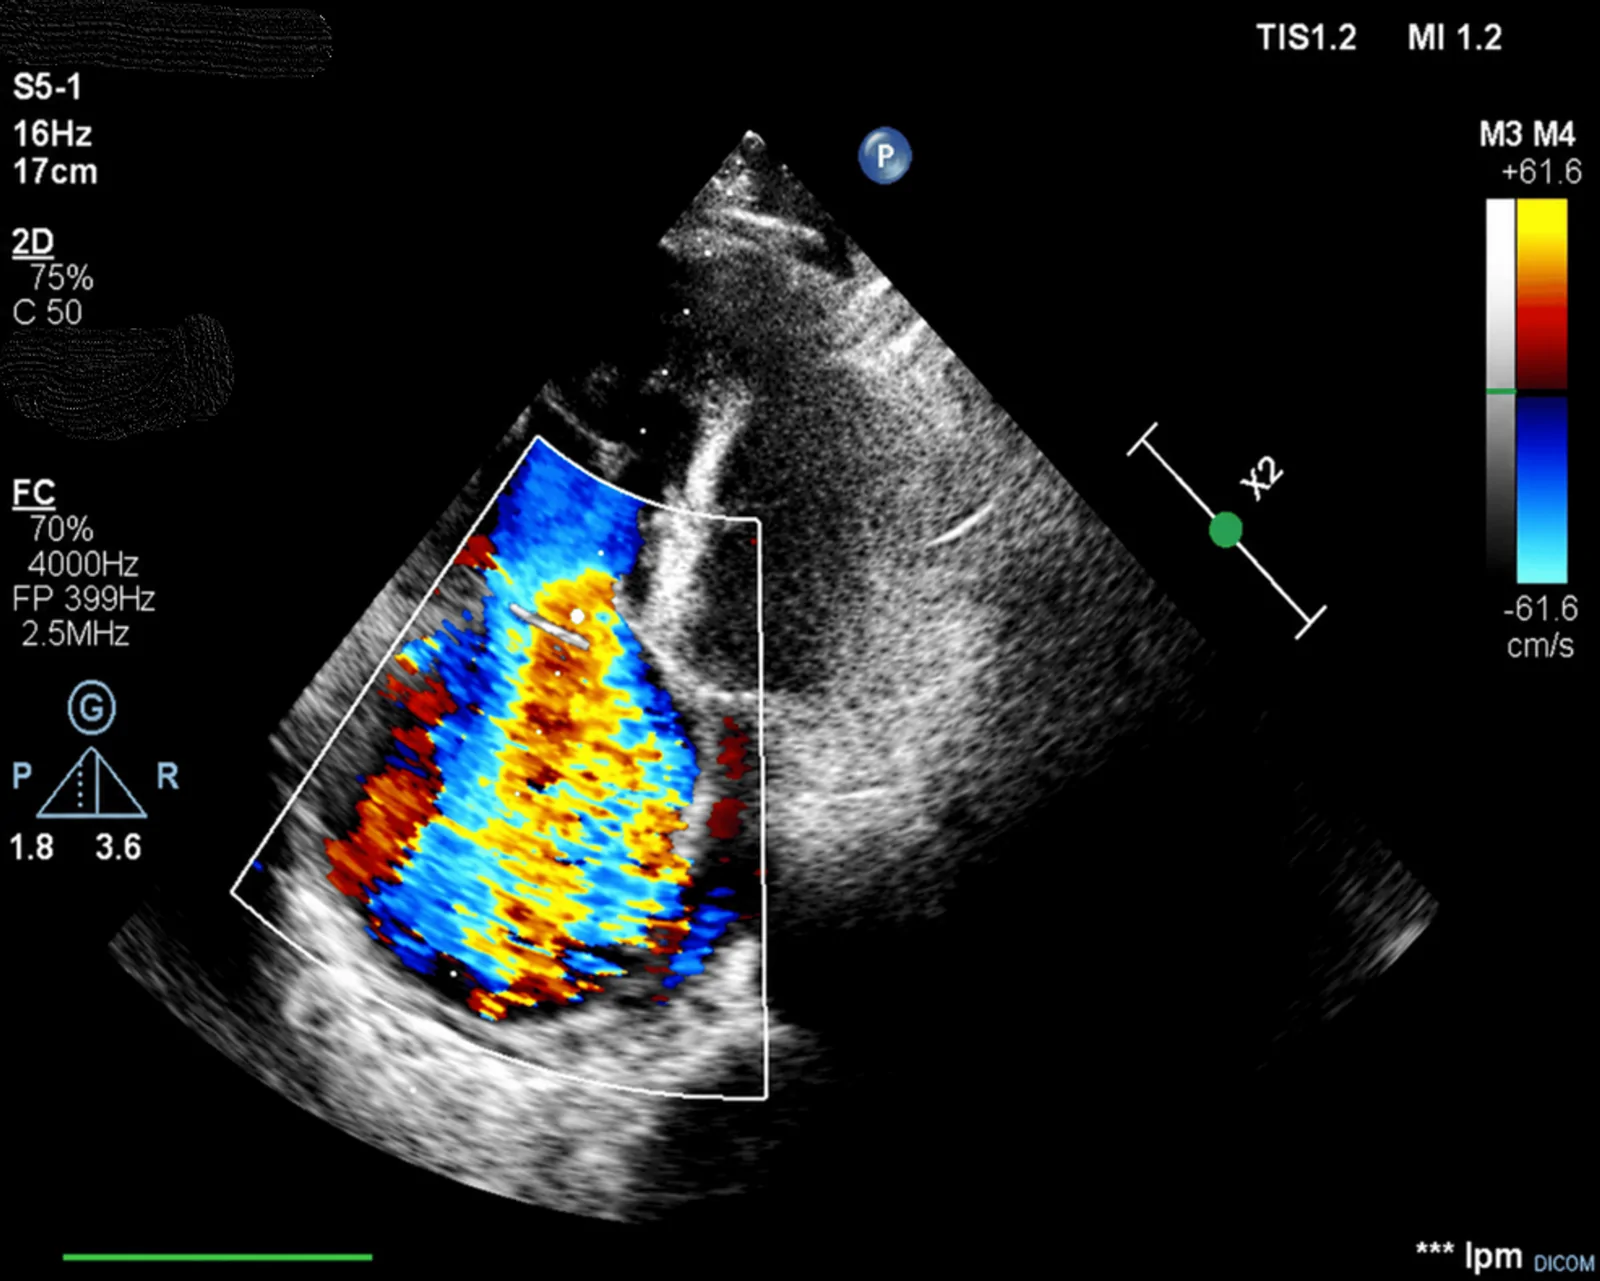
Transthoracic echocardiogram showing enlargement of the tricuspid annulus with tethered leaflets and a 16-mm coaptation gap, indicative of severe tricuspid regurgitation.
